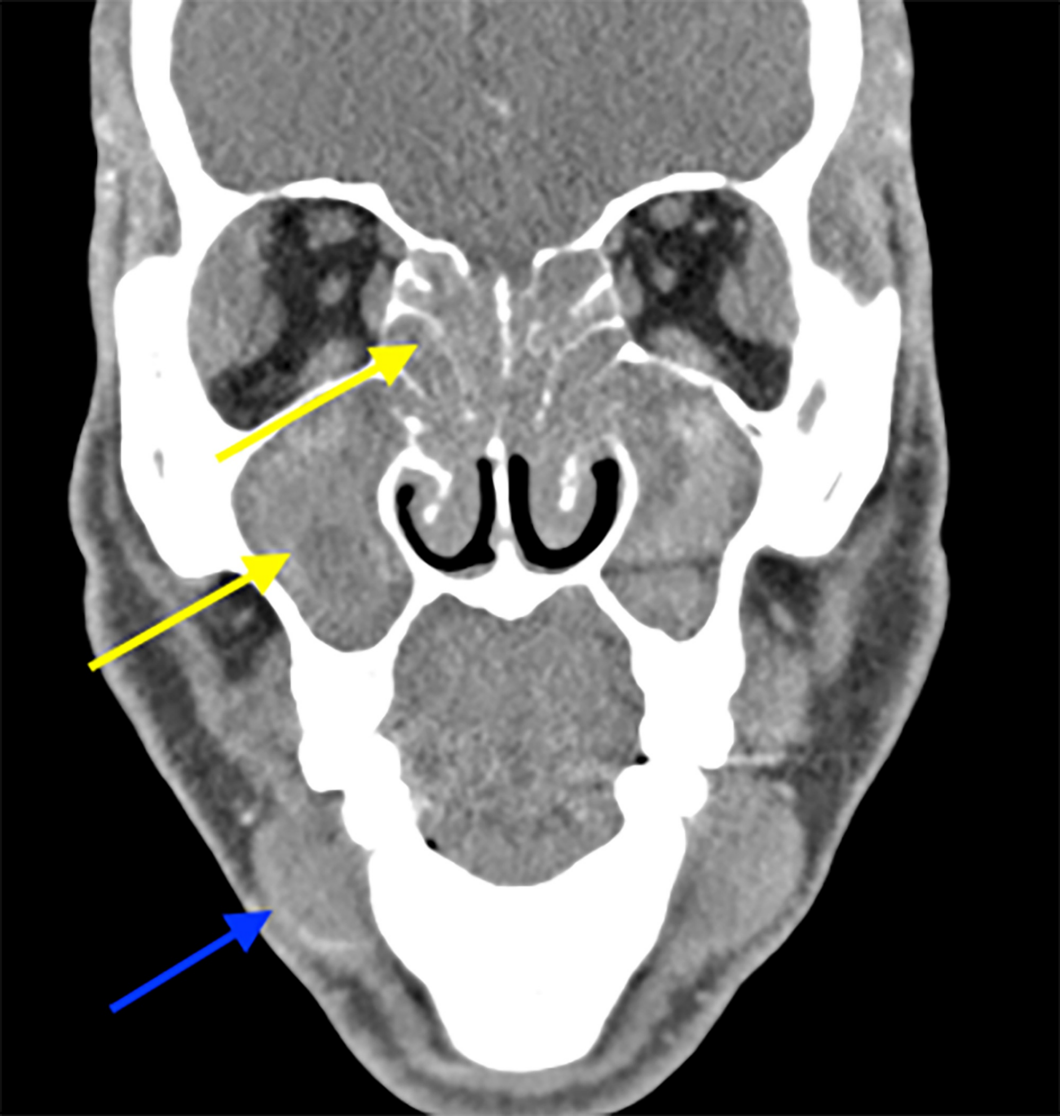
Coronal plane of computed tomography (CT) scan of the face demonstrating bilateral obliteration of ethmoid (upper yellow arrow) and maxillary sinuses (lower yellow arrow). Bilateral submandibular gland enlargement (blue arrow).
